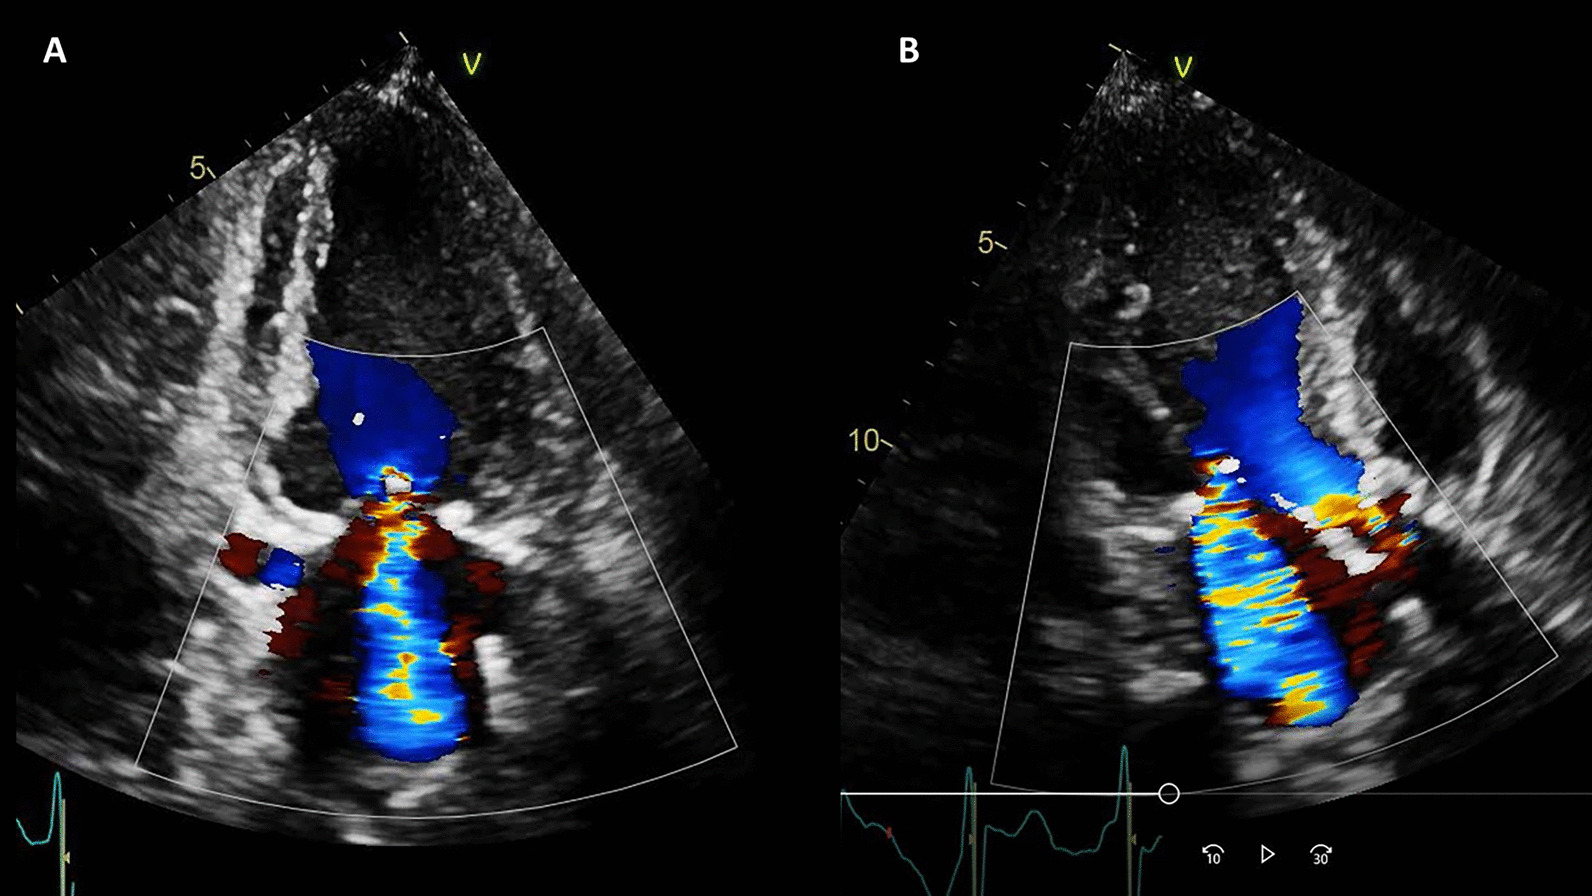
Case 4. Figure 2. Color Doppler echocardiography of A apical 2 chamber and B apical 3 chamber views. Severe mitral regurgitation and turbulent flow at the aortic valve
